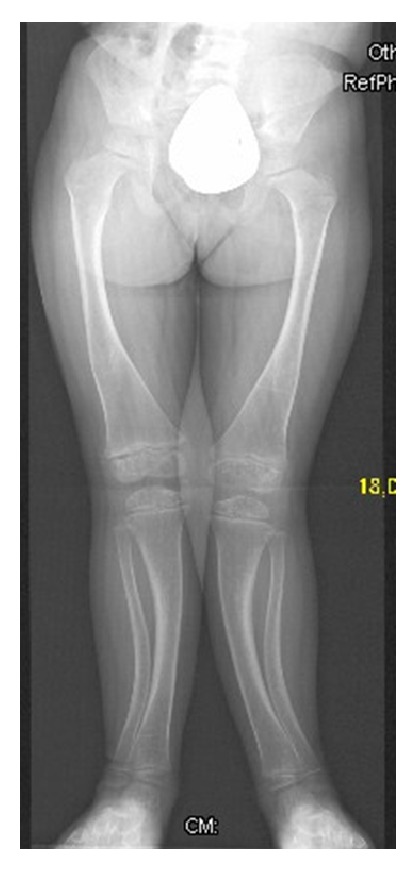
AP standing radiograph at the age of 7 years showed squaring of the iliac wings, coxa vara, and bilateral and symmetrical lateral bowing of the femora associated with thickening of the cortices. The tibiae and fibulae showed bilateral sabre deformity with posteromedial bowing between the middle and distal thirds of the tibia. Thickening of the tibial cortices was evident. The fibulae showed broadening/“tibialization” and posterior cortical thickening of the two bones.
